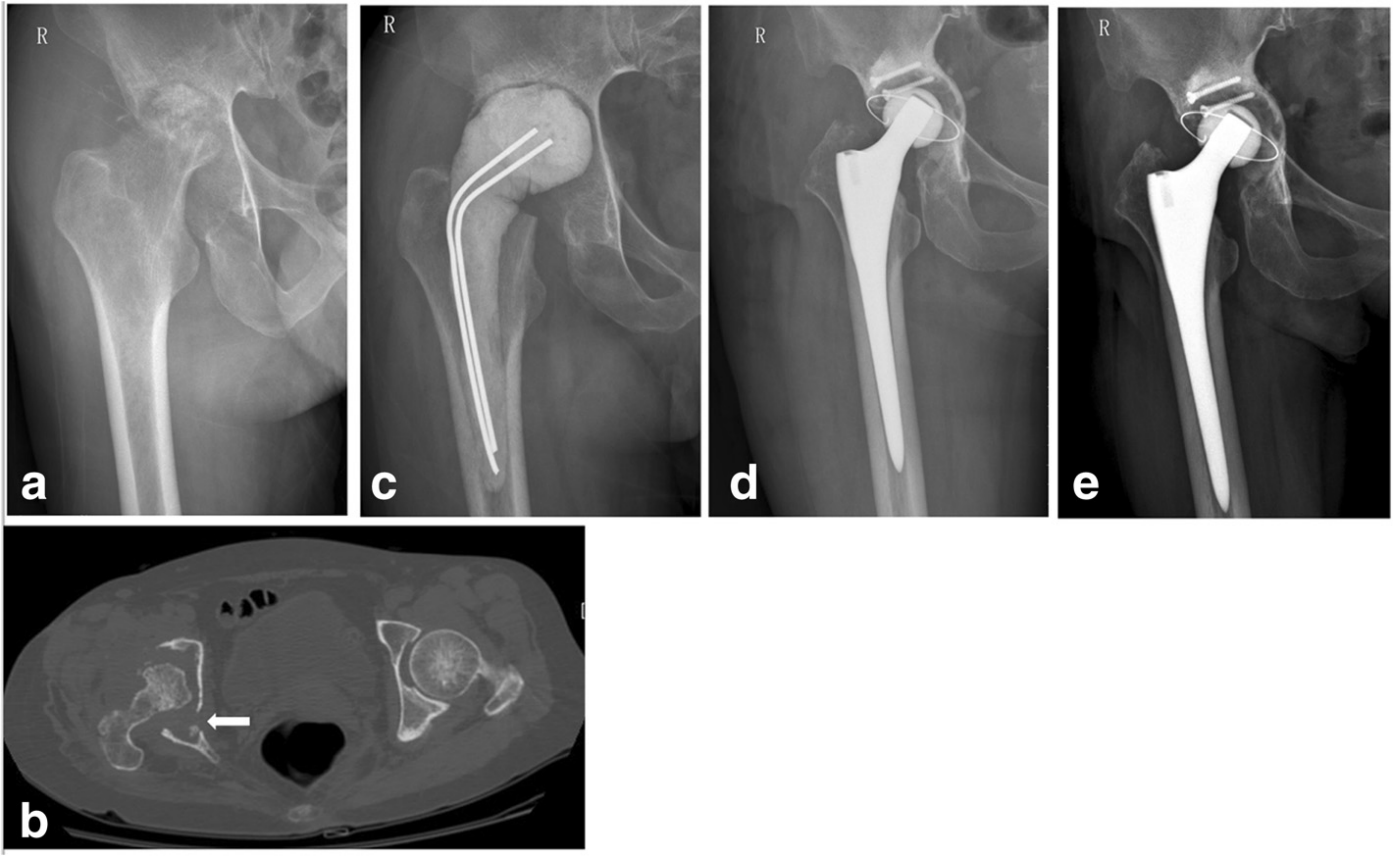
a A preoperative radiograph showing bony destruction and narrowing of the right hip joint space. b The CT scan showing bony destruction and a sinus tract to the pelvis (*white arrow*). c The femoral head was excised, and a cement spacer was planted in during the first operation. d The radiograph taken after the second operation showing bone graft fixed with two nails at the acetabular side. e The radiograph taken 4 years later showing no evidence of osteolysis or disease reactivation
